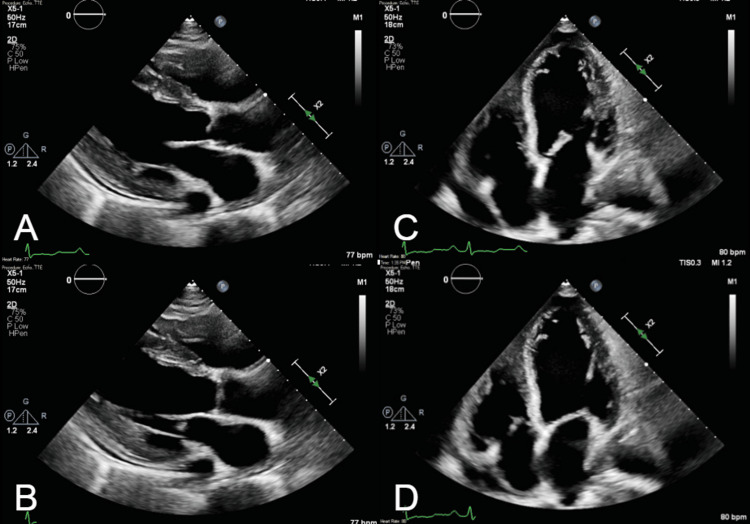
Parasternal long axis (A, B) and apical four-chamber (C, D) transthoracic echocardiography demonstrating a reduced ejection fraction of 20% with severe biatrial and biventricular dilatation.
